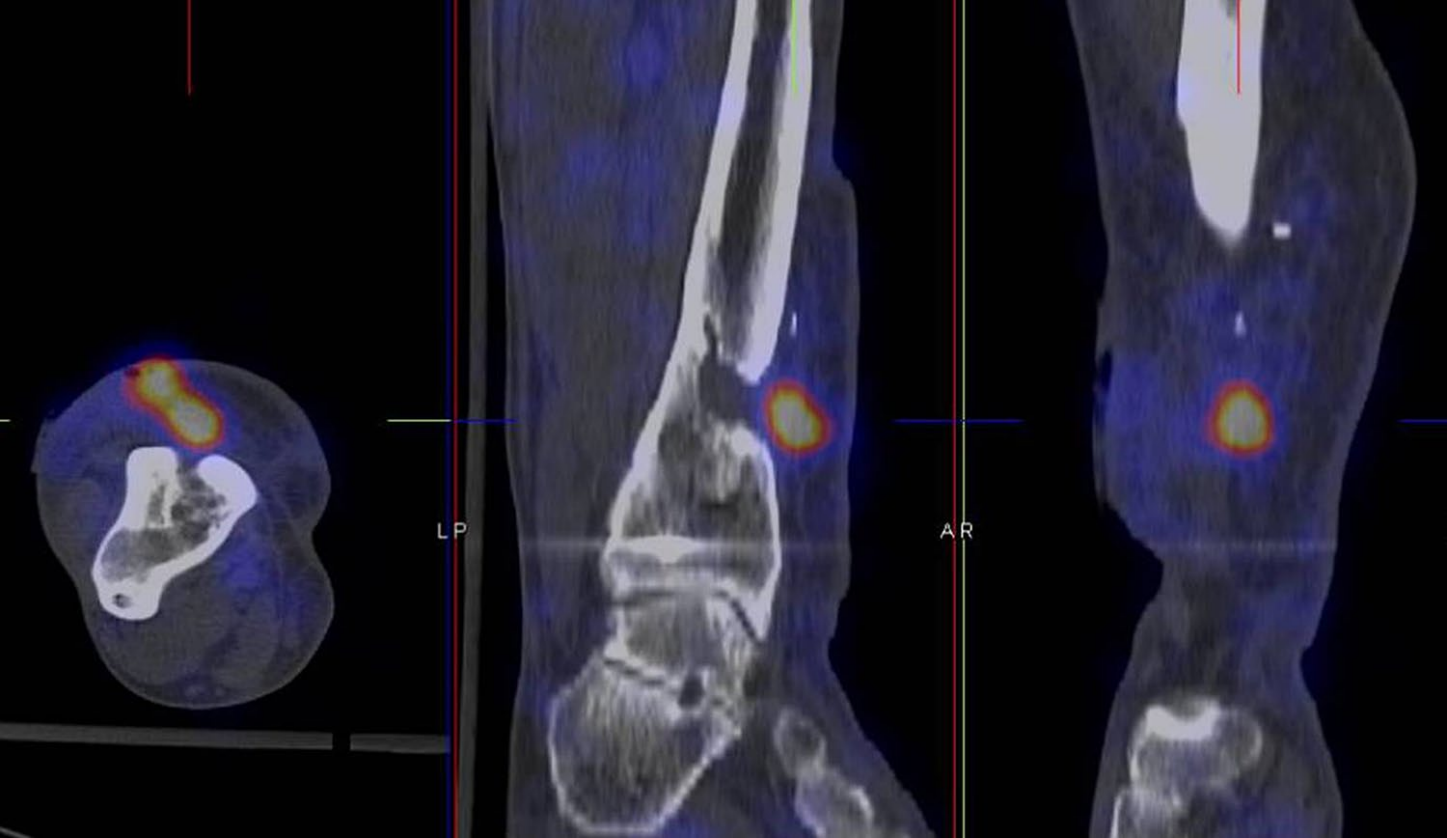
WBC scan SPECT-CT of patient A
